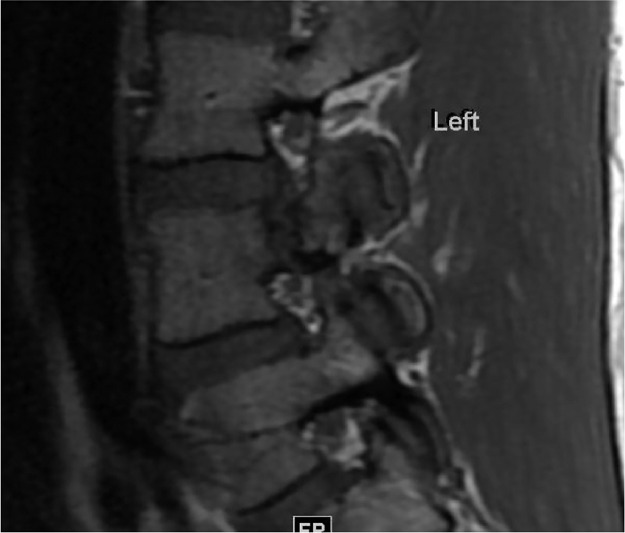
Sagittal T1 weighted MRI showing left pedicle fracture L4. Again, no marrow fat signal loss to suggest bone edema.
